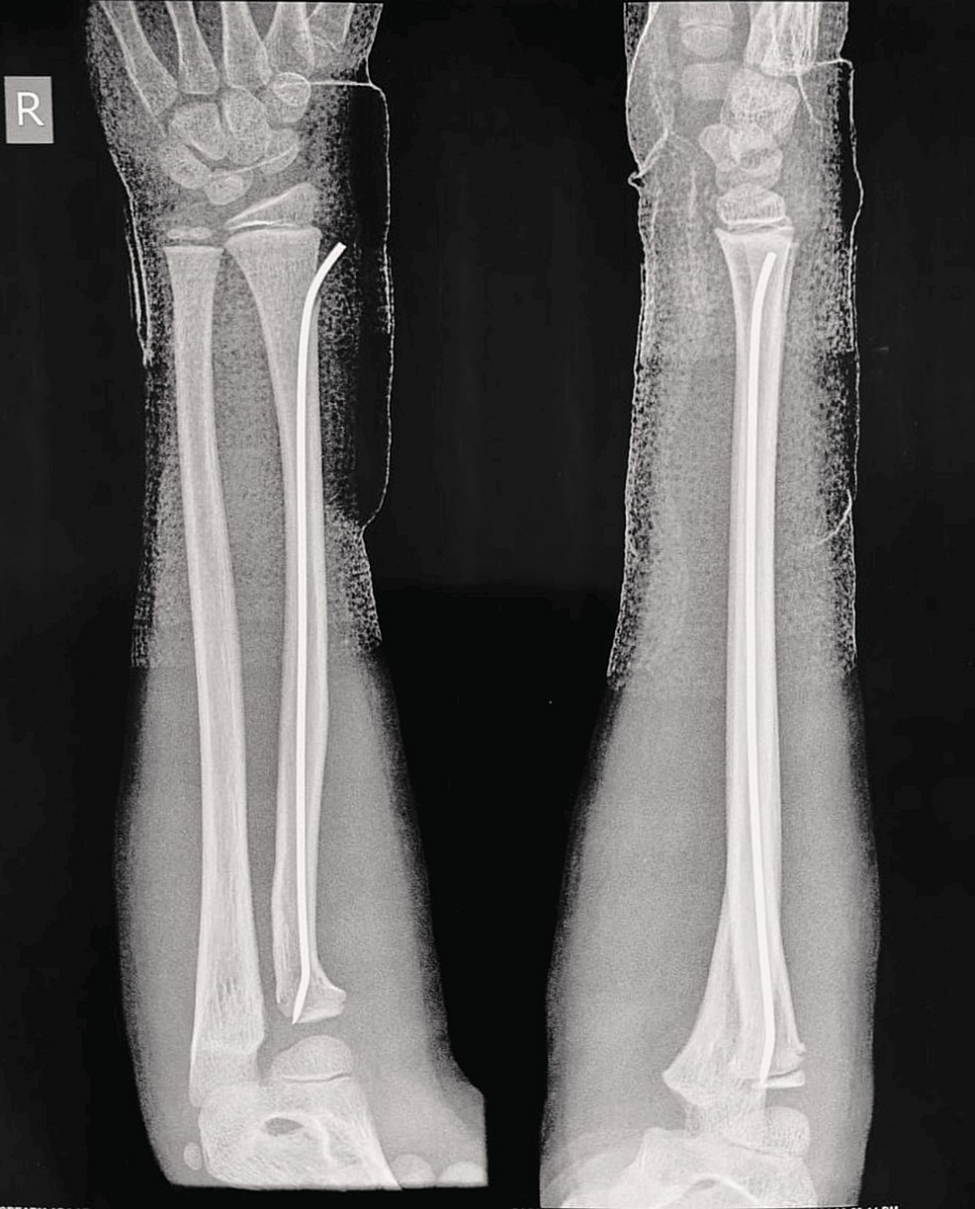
Postoperative anteroposterior and lateral radiographs following the Metaizeau procedure
